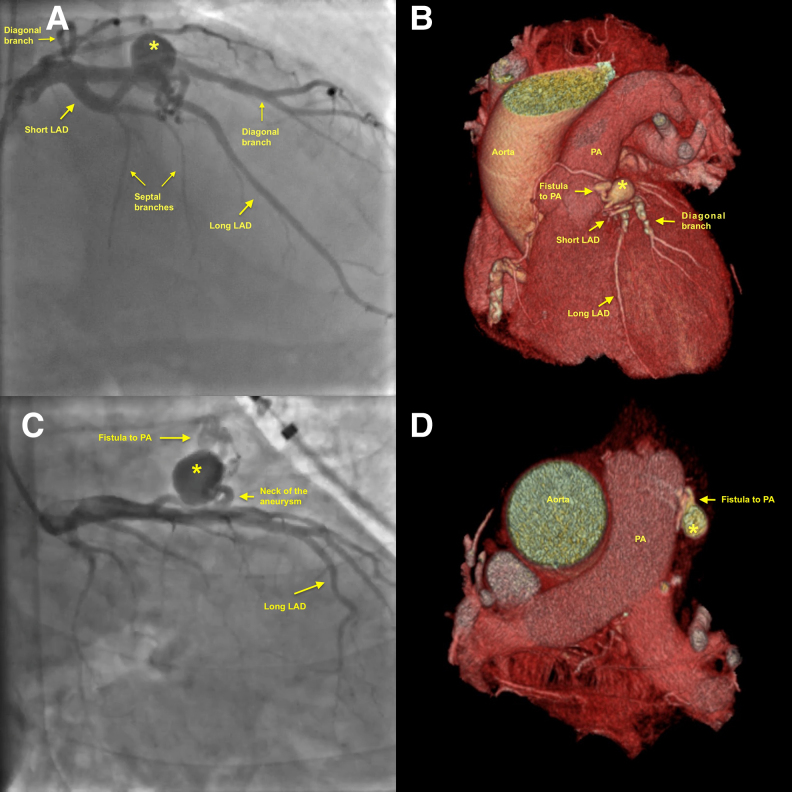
Angiographic images showing the short and long left anterior descending arteries and the aneurysm from the right anterior oblique cranial projection (*A*) with matching 3D computed tomography angiographic view showing the same structures (*B*). Right anterior oblique caudal projection allowed better visualization of the aneurysm neck, aneurysmal sac, and fistula to the pulmonary artery (*C*). Horizontal cross-sections at the level of pulmonary artery show matching images of fistula and aneurysm on T angiography (*D*). Asterisk shows the aneurysmal sac. LAD, left anterior descending artery; PA, pulmonary artery.
